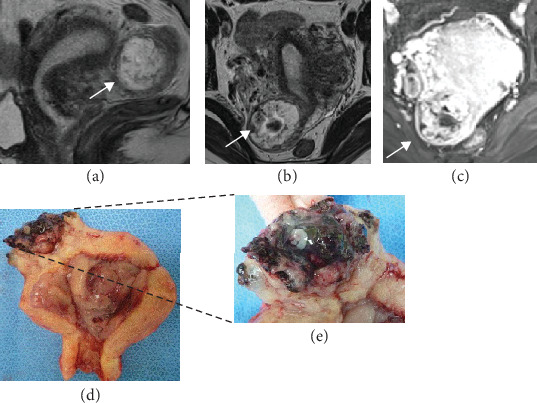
MR image performed at 7 weeks and 5 days in case 1 shows an interstitial pregnancy. A heterogeneously-enhanced hypervascular mass is identified on the right side of the uterine fundus. The mass measures 52 mm, which is larger than a GS at the corresponding estimated gestational age. Arrows indicate the pregnancy-associated lesion. (a) A sagittal T2-weighted MR image. (b) An axial T2-weighted MR image. (c) An enhanced axial MR image. (d) Gross pathology of the uterus and the pregnancy-related tissue. (e) An enlarged view of the implantation site.
